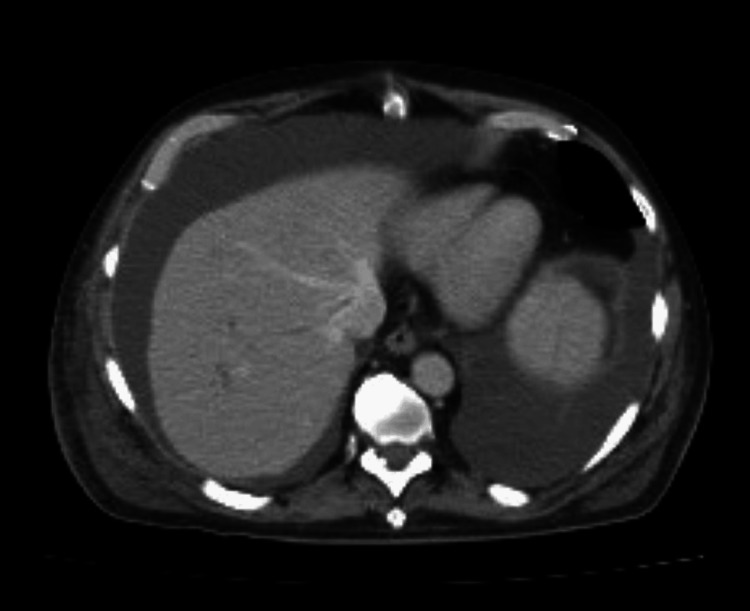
Axial CT of the abdomen and pelvis demonstrating large-volume ascites
